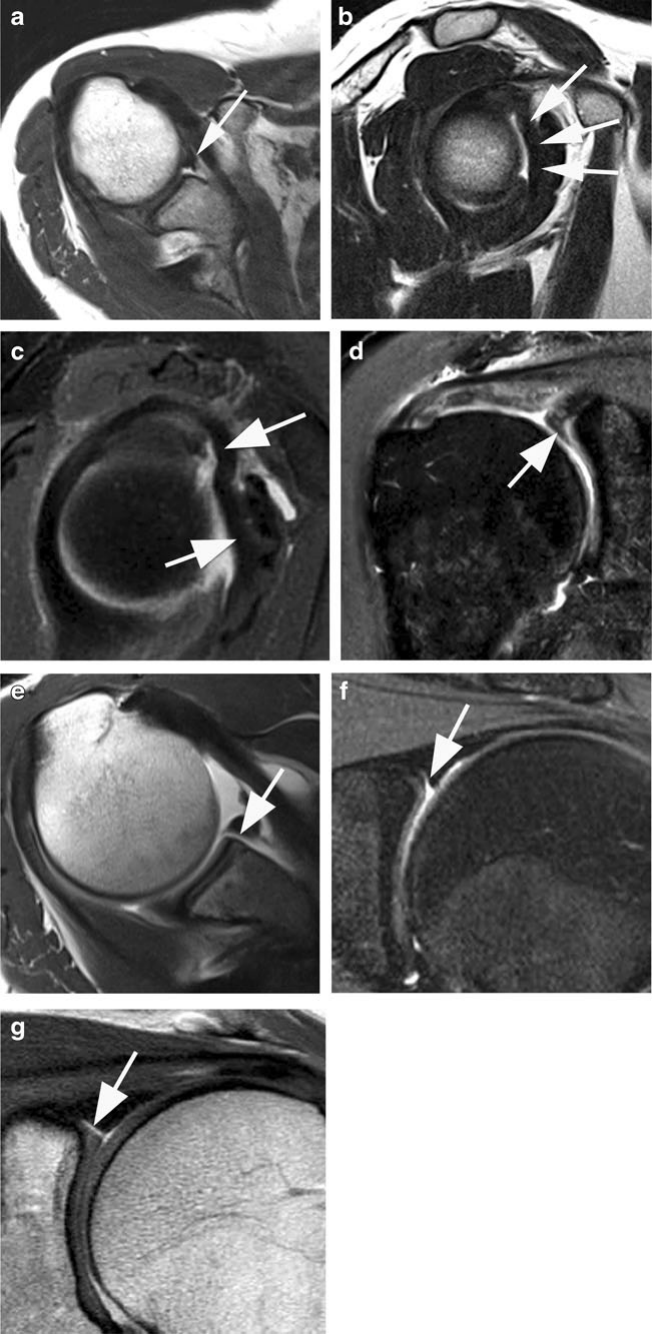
Normal variants around the superior labrum and the biceps anchor. a, b Axial (a) and sagittal (b) non fat-suppressed proton density-weighted images show a missing anterior superior labrum. The middle gleno-humeral ligament appears thick and of cord-like appearance representative of a so-called Buford-complex (*arrows*). c Another example of a Buford complex on a fat-suppressed proton density-weighted image *(arrows)*. d Coronal fat-suppressed proton-density-weighted image shows a meniscoid shaped labrum (*arrow*). e Axial T1-weighted MR arthrography image shows a so-called sublabral foramen (*arrow*). f, g The attachment of the biceps tendon at the glenoid may show different appearances. Proton density-weighted fat suppressed (f) and nonfat-suppressed (g) images of two different patients show a so-called Type II bicipito-labral complex representing a recess at the labral attachment (*arrows*). Such recesses point medially and as such may be distinguished from so-called SLAP II lesions that are commonly pointing towards the lateral aspect of the biceps anchor/proximal tendon
